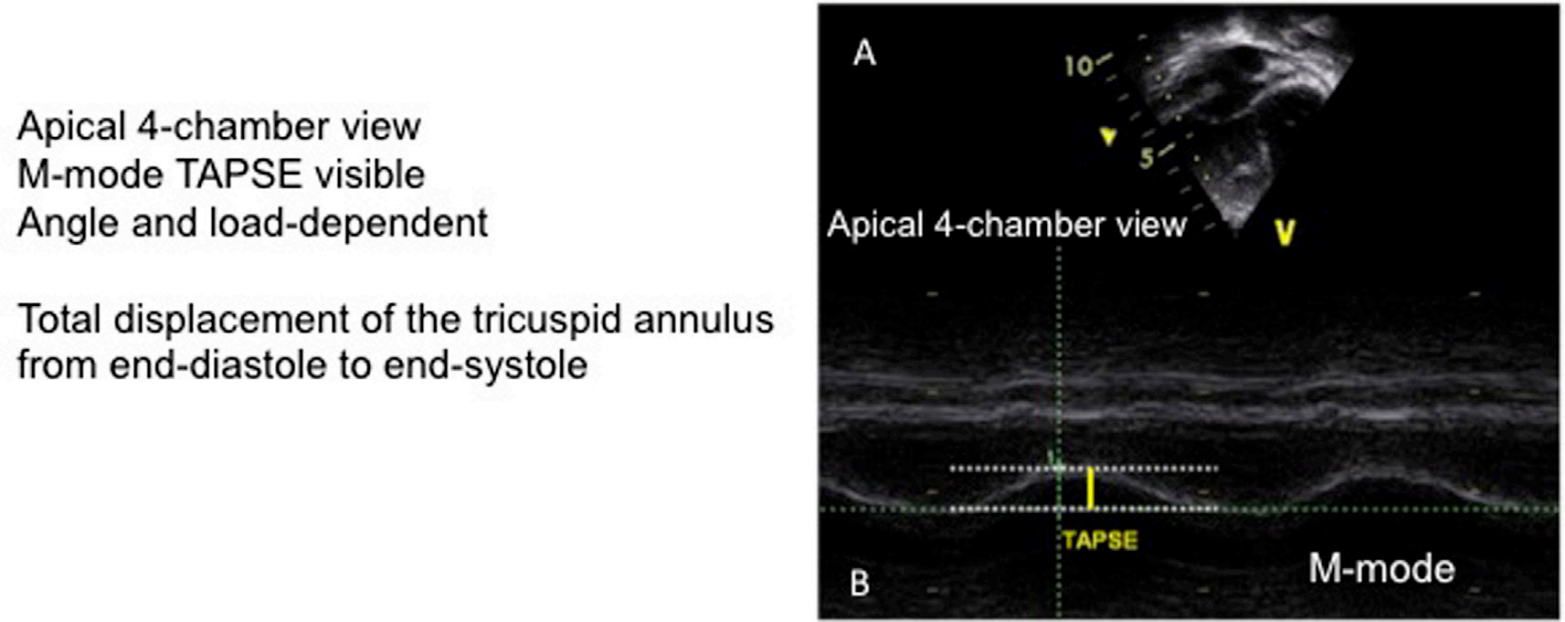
Tricuspid annular plane systolic excursion (TAPSE). (A) Four-chamber view of the right ventricle with ultrasound beam aligned on the tricuspid annulus. (B) Measurement of the TAPSE on the M mode image.
